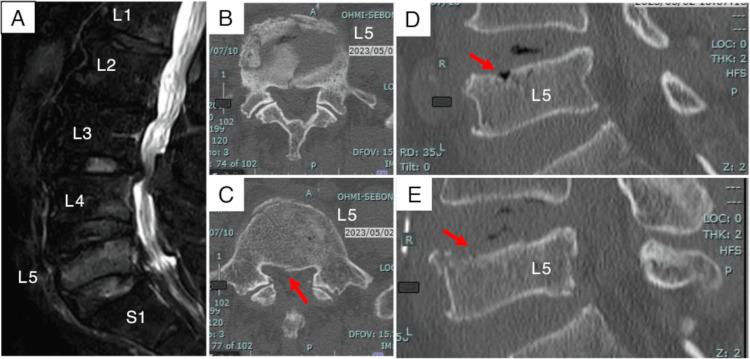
Initial radiological findings (A) A sagittal short tau inversion recovery image shows a high-intensity lesion in the L5 vertebral body. (B, C) Axial computed tomography images demonstrate an upper vertebral endplate defect and a posterior wall fracture (red arrow). (D, E) Sagittal computed tomography images confirm the upper vertebral endplate defect.
